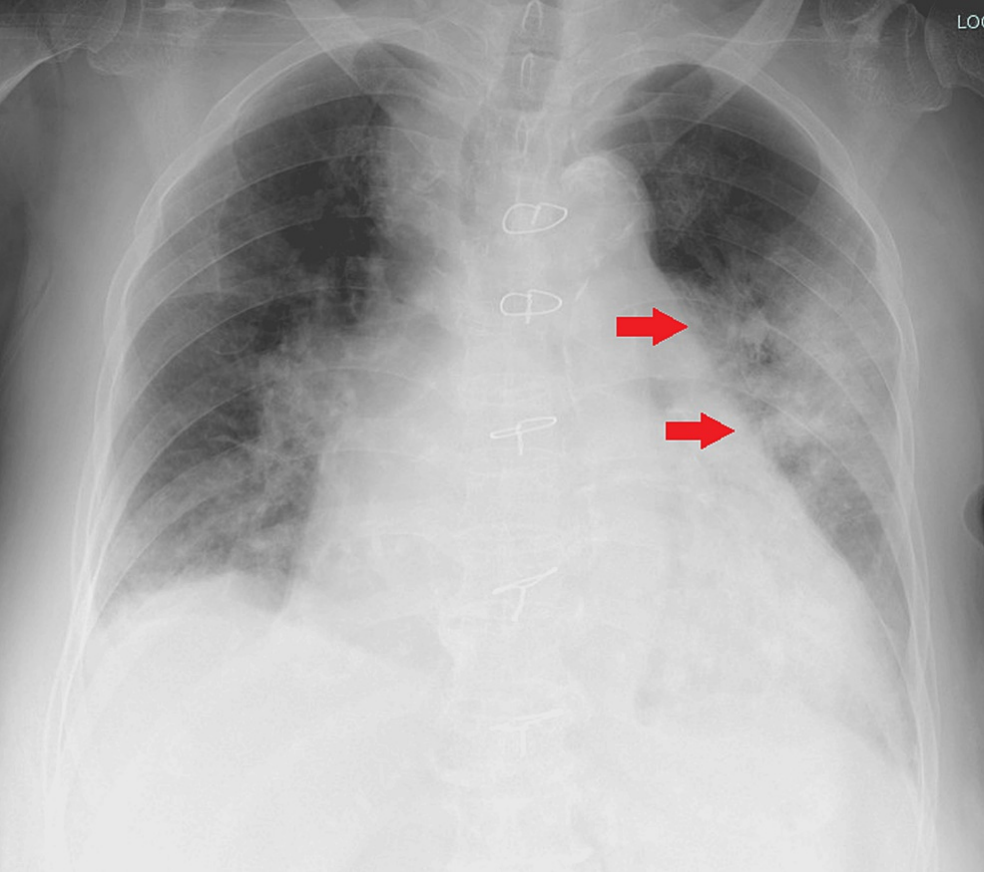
Chest X-ray on hospital admission in case 1. A localized infiltration (red arrows) was noted in the left upper lobe.
